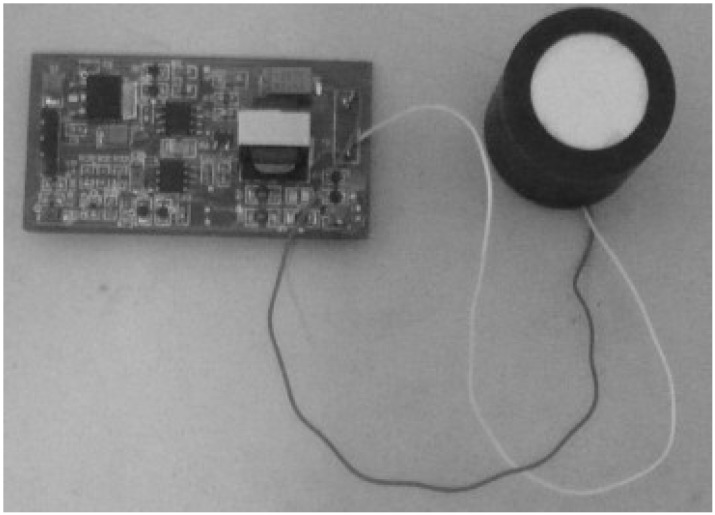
The physical connection of the ultrasonic sensor with the control board for TRV identification experiments.
